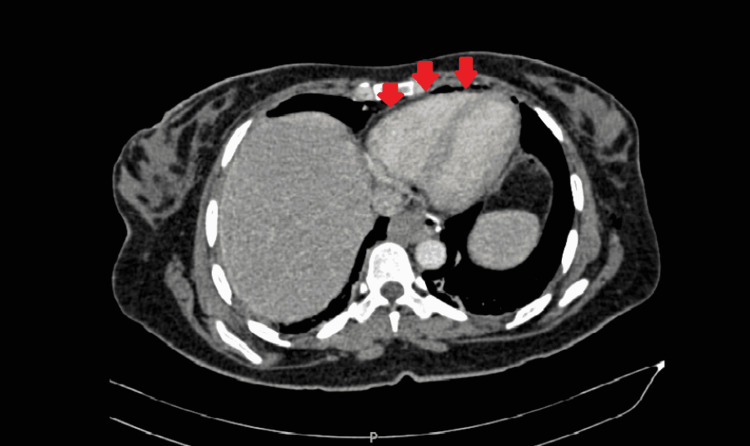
Chest CT Scan Showing Pneumomediastinum Red arrows pointing to rim of air are seen within the mediastinum. CT, computed tomography.
